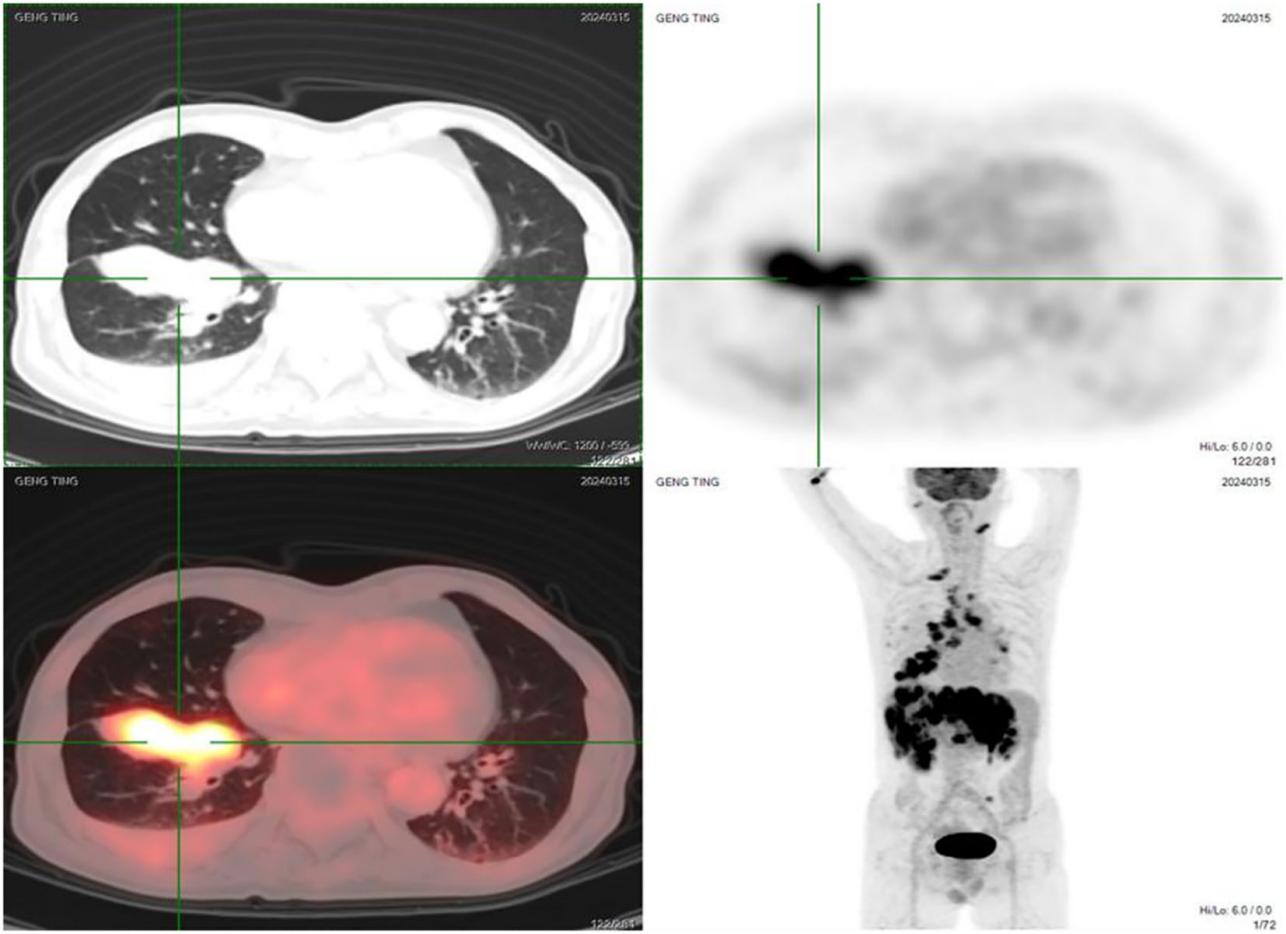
Pre-treatment PET/CT images (March 15, 2024) showing the primary lesion in the right lower lobe, multiple bilateral pulmonary metastases, extensive lymph node metastases, brain metastases, liver metastases, and bone metastases.
